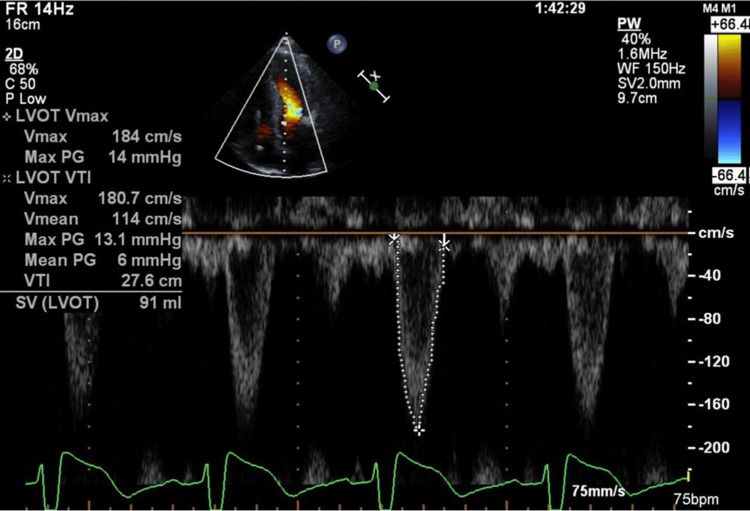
Echocardiography before the third pregnancy The left ventricular outflow tract pressure gradient had improved to 6-13 mmHg.
